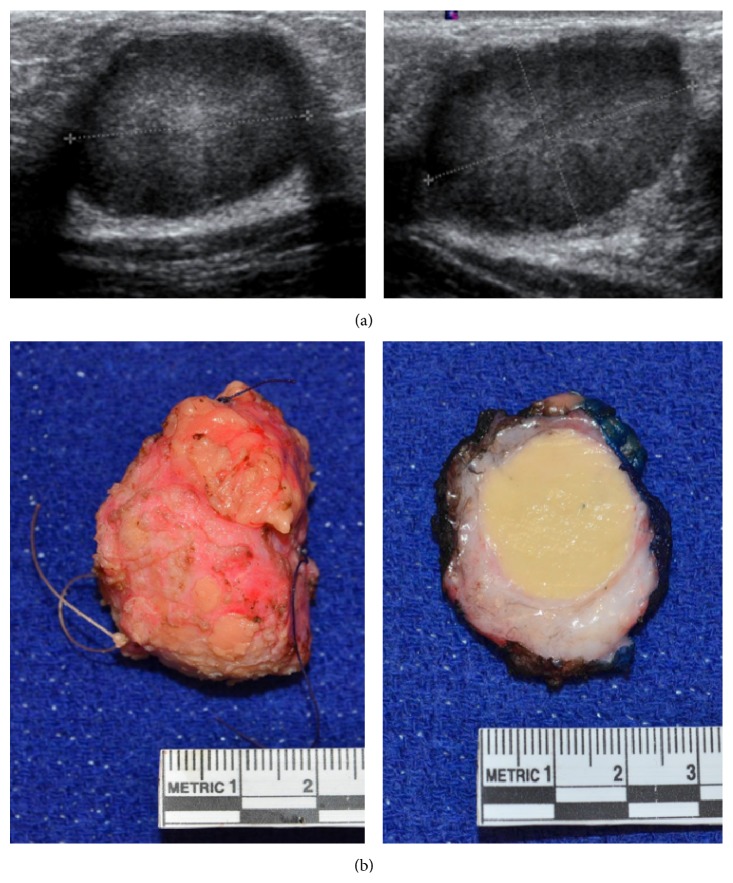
(a) Ultrasound images of a granular cell tumor of the breast showing a solitary mass without vascularity. (b) Gross pathologic images of the resected granular cell breast tumor.
